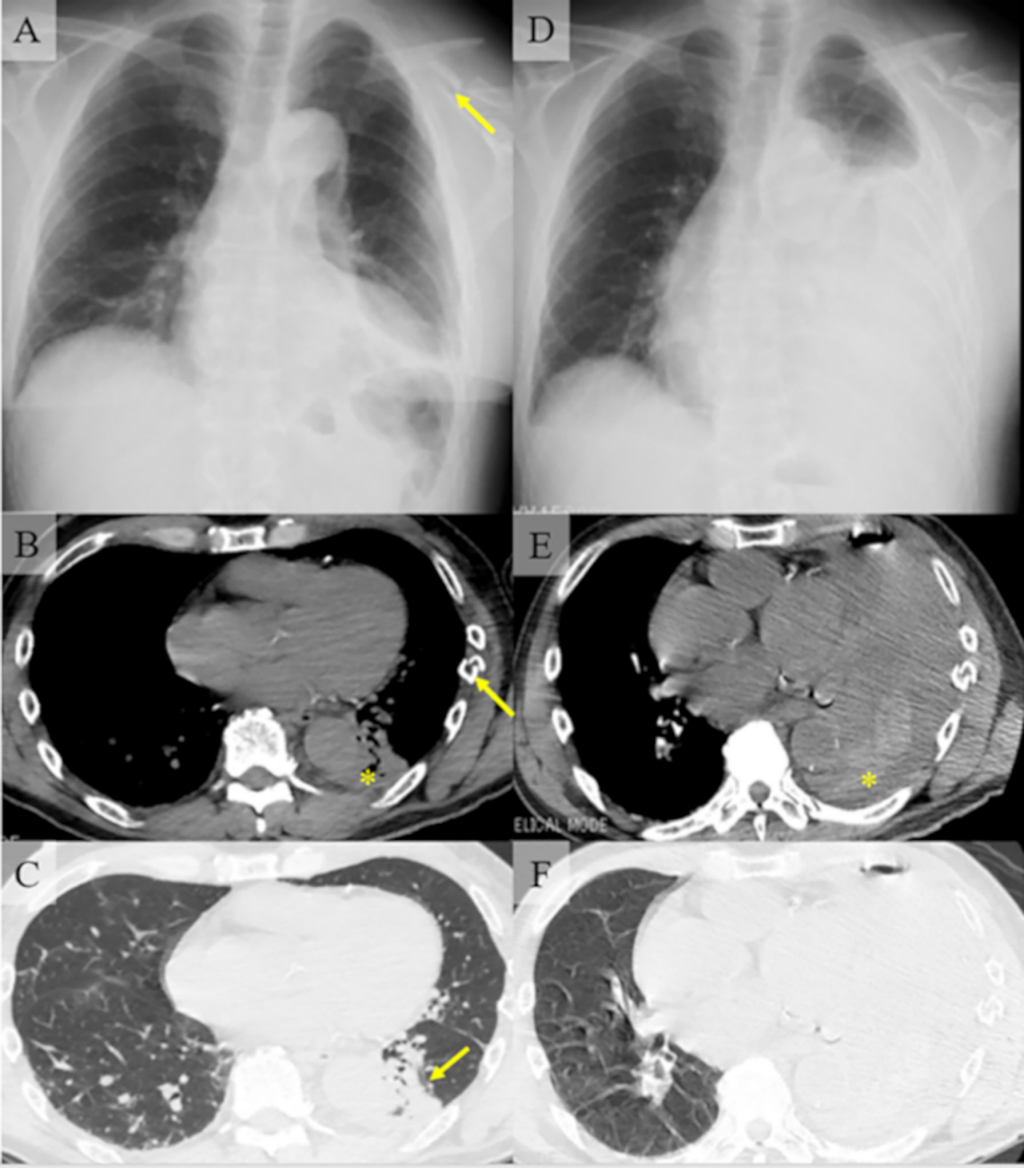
Radiological images of the patient at first visit (A–C). (A) Chest x‐ray revealed multiple rib fractures, a small amount of left pleural effusion, and a fracture of the left clavicle (arrow). (B) Chest computed tomography revealed fractures of the left 2nd to 8th ribs. This figure focused on the fracture of the 7th rib (arrow). A small amount of high‐density pleural effusion was also revealed (*). (C) A small contusion of the left lower lobe was revealed (arrow). Radiological images after vital signs changed (D–F). (D): A massive left pleural effusion was found. (E) A massive high‐density left pleural effusion was also observed (*). (F) Lung window setting of panel E.
